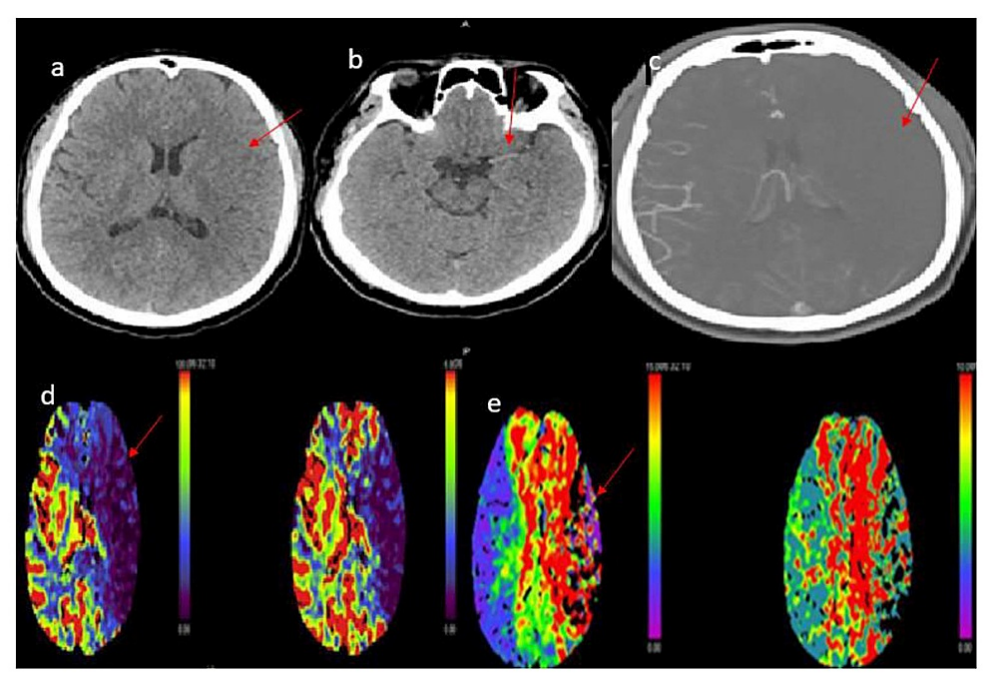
CT head, perfusion and angiography showed left acute infarct of MCA and left capsule-ganglionic region with matched perfusion defect, and right paramedian frontal and parietal lobes showed penumbra (d-e), left MCA dense sign and no collateral vessels visible to the left MCA ischemic site (a-c). MCA: Middle cerebral artery
